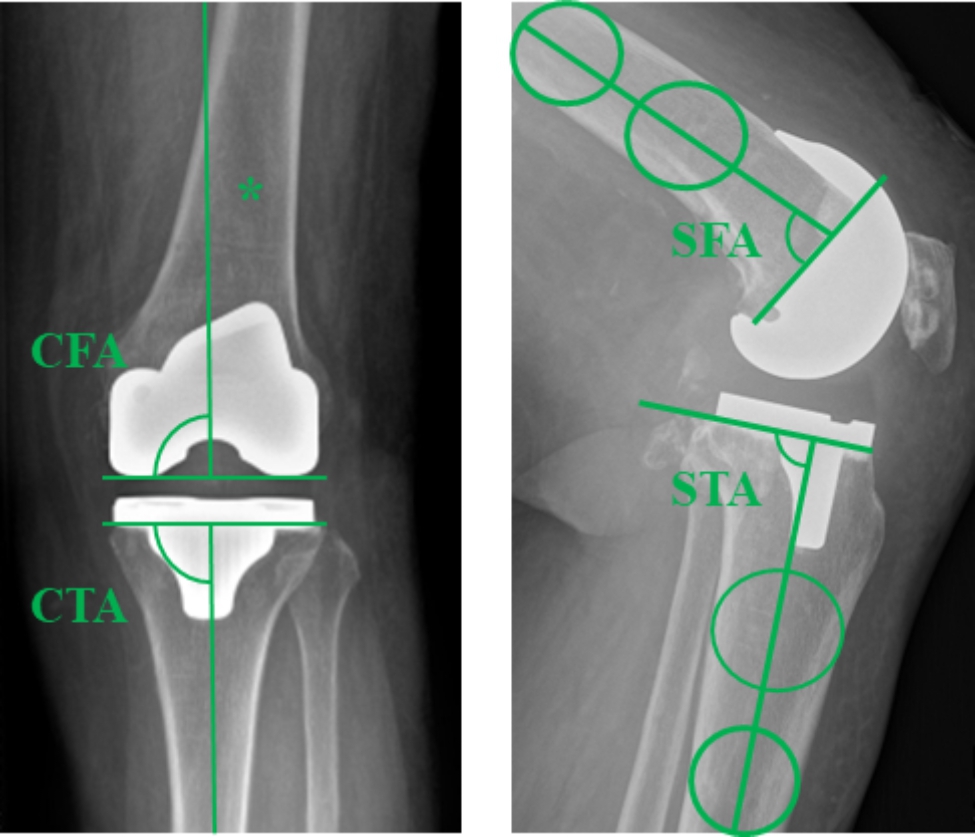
Component position angle on radiograpy. CFA was determined as the medial angle between the line connecting the femoral component condyles and the mechanical axis of the femur. CTA was defined as the medial angle between the horizontal tibial tray and the mechanical axis of the tibia. SFA was the proximal angle between the line perpendicular to the distal cement surface and the anatomical axis of the femur. STA was defined as the angle between the axis of the horizontal tibial tray and the anatomical axis of the tibia ^*^CFA, coronal femoral alignment; CTA, coronal tibial alignment; SFA, sagittal femoral alignment; STA, sagittal tibial alignment
